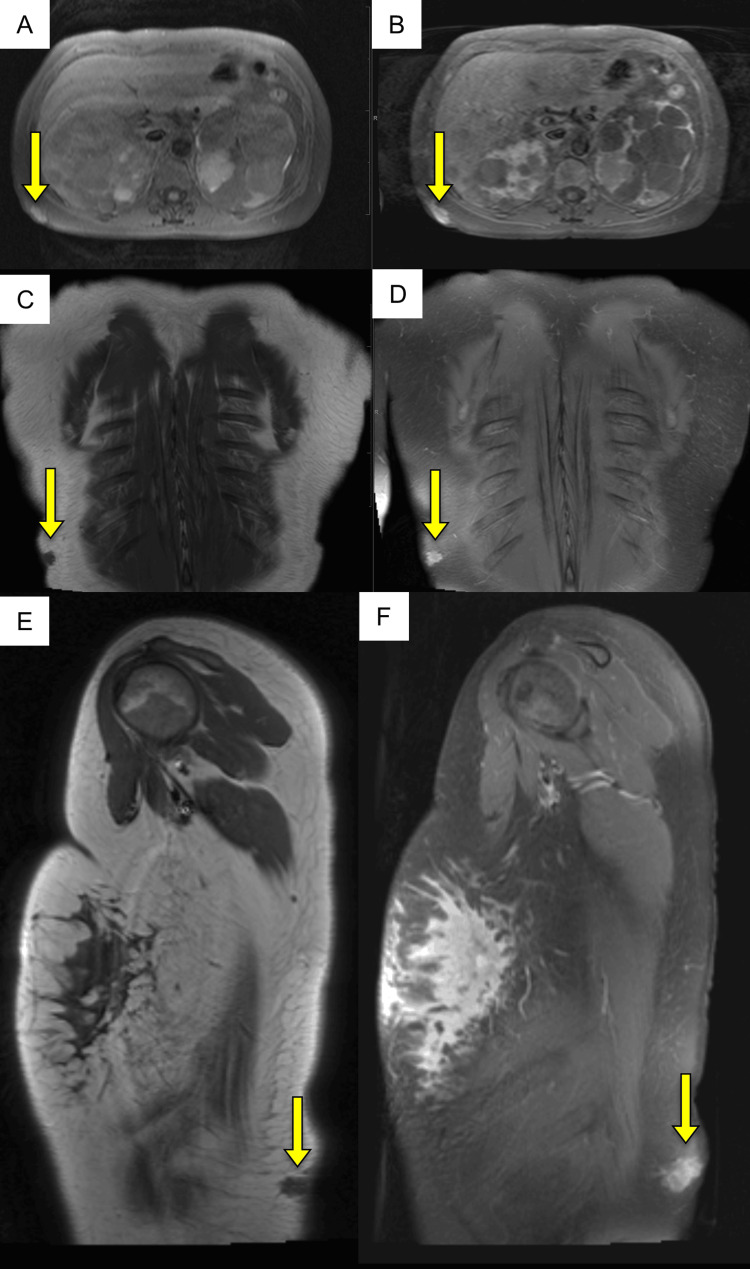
Multiplanar MRI of the thorax demonstrating a right posterolateral chest wall mass (yellow arrows). (A, C, E) Pre-contrast T1-weighted images in axial (A), coronal (C), and sagittal (E) planes show a well-defined chest wall lesion isointense to muscle. (B, D, F) Corresponding post-contrast fat-suppressed T1-weighted images in axial (B), coronal (D), and sagittal (F) planes reveal heterogeneous enhancement of the mass.
